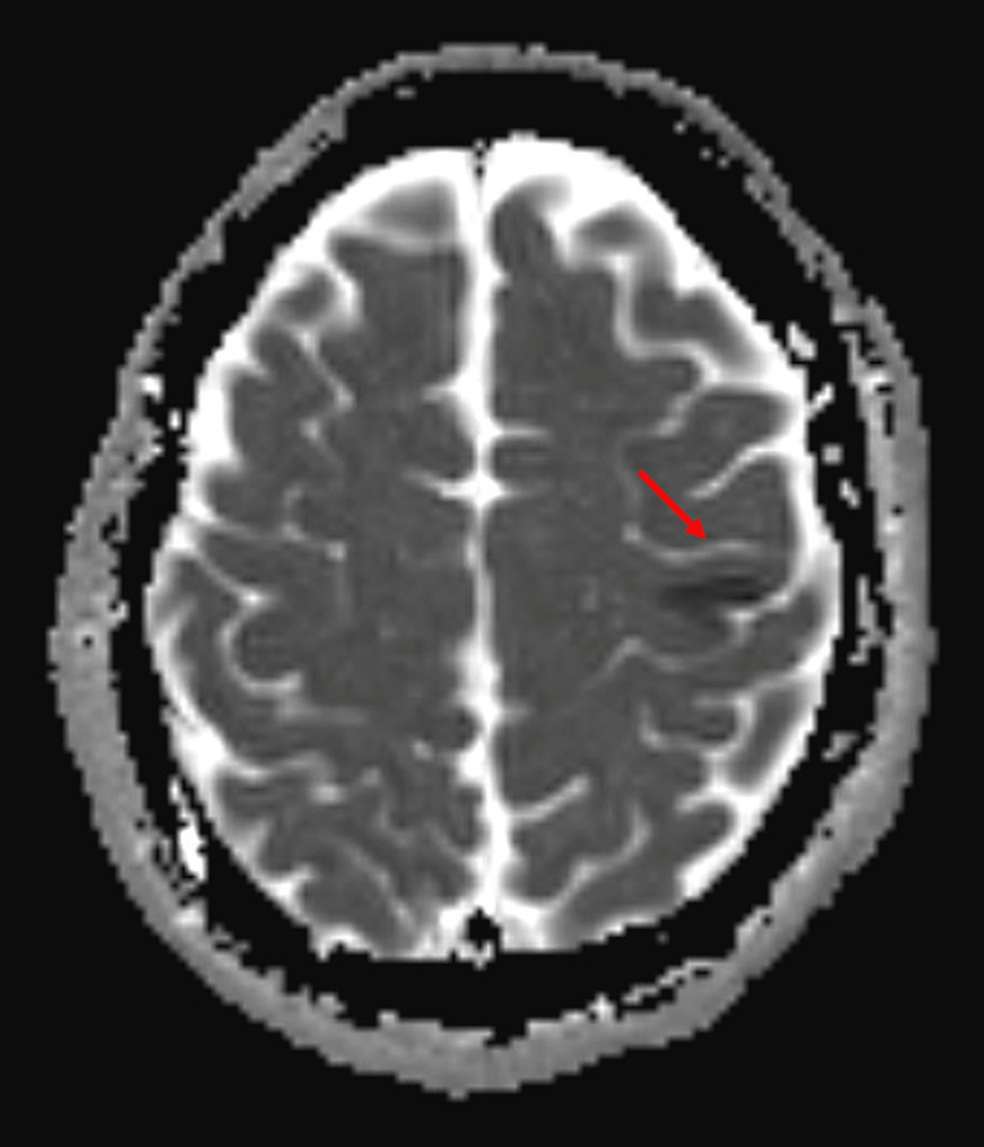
The ADC MRI image shows restricted diffusion with corresponding low ADC signal at the lateral portion of the primary motor cortex indicating hyperacute ischemic infarct (Red Arrow). ADC: apparent diffusion coefficient
